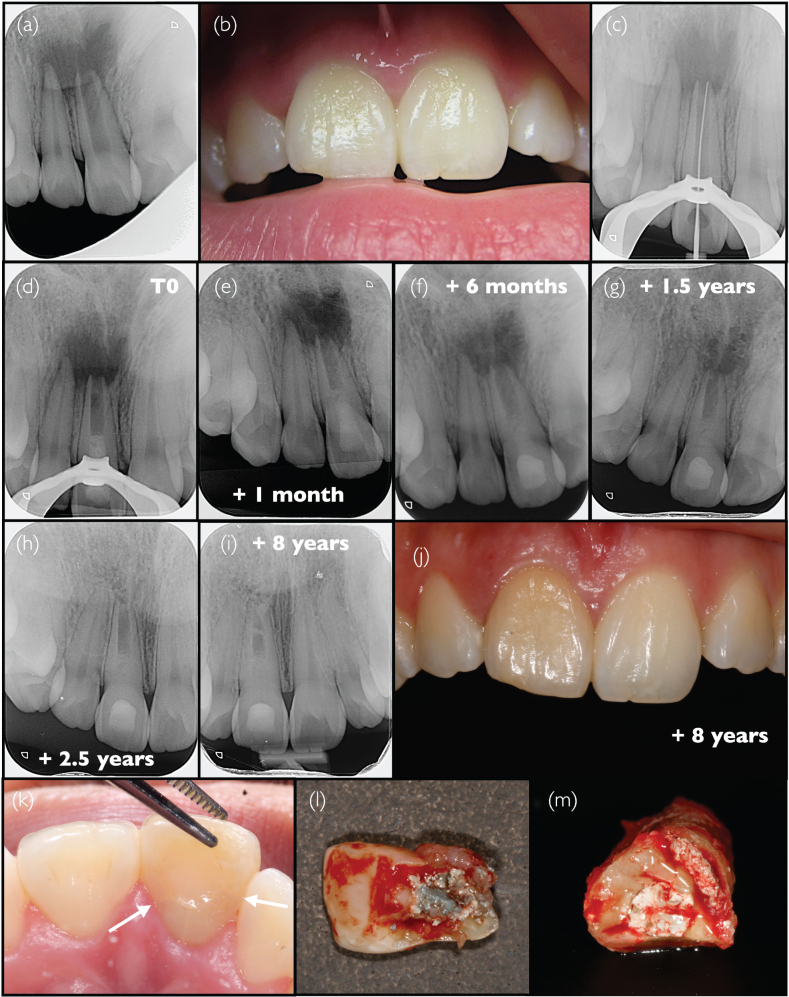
Clinical Case #3 (A) Pre-operative radiograph and (B) intraoral photograph. (C) Intraoperative radiograph showing working length determination and (D) radiograph after completing the revitalisation procedure. (E–J) Post-operative follow-up radiographs taken periodically, from 1 month to 8 years after the procedure. (I) Radiograph obtained during an emergency visit 8 years post-treatment, showing (J) an intraoral photograph and (K) a corono-radicular fracture originating from the palatal side. (L) Image of the extracted crown fragment and (M) the fracture interface.
